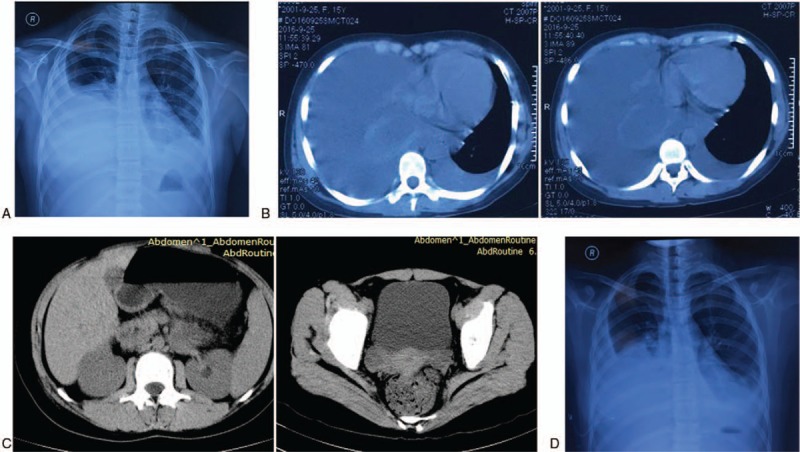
Radiographic examination at the second Affiliated Hospital of Nanjing Medical University. (A, B) X-ray and computed tomography (CT) of the chest showed massive bilateral pleural effusion, more prominent on the right side. (C) CT of the whole abdomen demonstrated a mass in the body of the pancreas and nodules in the bilateral adnexal regions. (D) Repeat chest x-ray showed finding similar to the previous x-ray (September 25, 2016).
